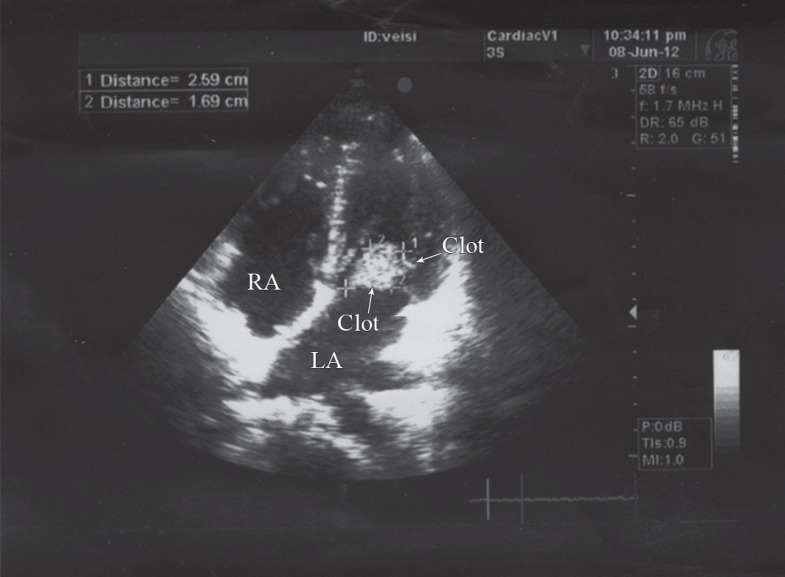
Transthoracic echocardiography in the four-chamber view, showing a large clot in the left atrium, attached via a long stalk to the residual resected interatrial septum (arrows)
